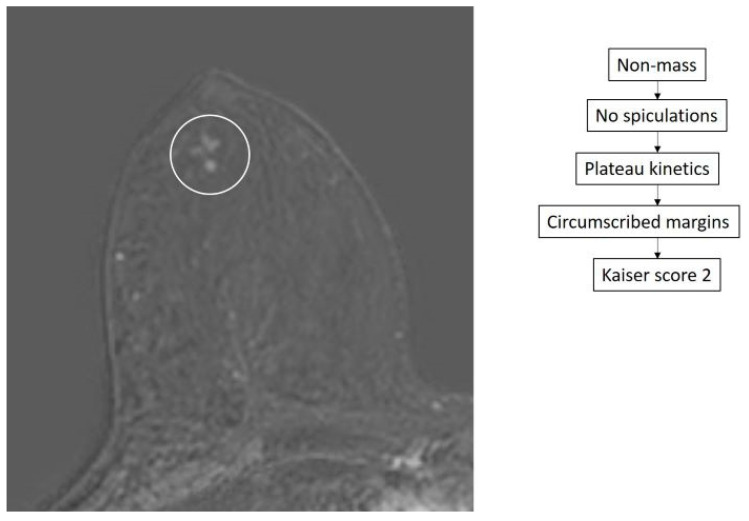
True negative case of a 47-year-old patient with a recent diagnosis of left breast cancer. Staging breast MRI shows clumped non-mass enhancement (circle) in the contralateral breast with a Kaiser score of 2. MRI guided biopsy was performed yielding proliferative fibrocystic changes.
